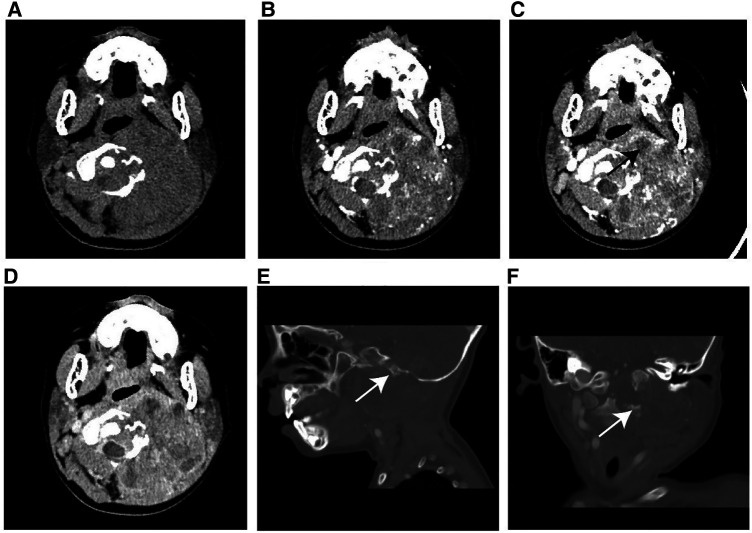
Typical instance of soft tissue RT in a female infant of 36 months (clinical manifestations: left neck swelling and pain for a month). (A) Non-enhanced CT scans revealed a moderately hypodense mass. (B–D) On multi-phase enhancement, the density of the tumor was higher than that of the adjacent muscle layer, with CT values for each period being around 56HU, 64HU, and 82HU, respectively. It showed heterogeneous enhancement and peripheral cyst (arrow). (E,F) Sagittal and coronal images revealed bone destruction in the adjacent skull base and multiple cervical vertebrae (arrow).
